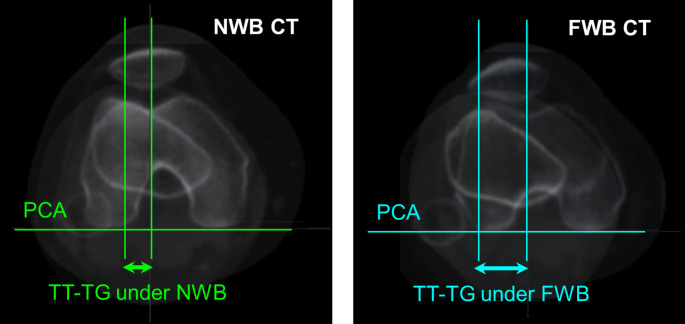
Axial CT comparison of the tibial tubercle–trochlear groove (TT–TG) distance under non-weight-bearing (NWB, left) and full-weight-bearing (FWB, right) conditions.The TT–TG distance increases from 12.3 ± 4.7 mm under NWB (green arrow) to 20.3 ± 3.9 mm under FWB (blue arrow) (*p* < 0.001), exceeding the conventional pathological threshold of 20 mm and potentially influencing indications for distal realignment in medial patellofemoral ligament reconstruction. PCA: posterior condylar axis
